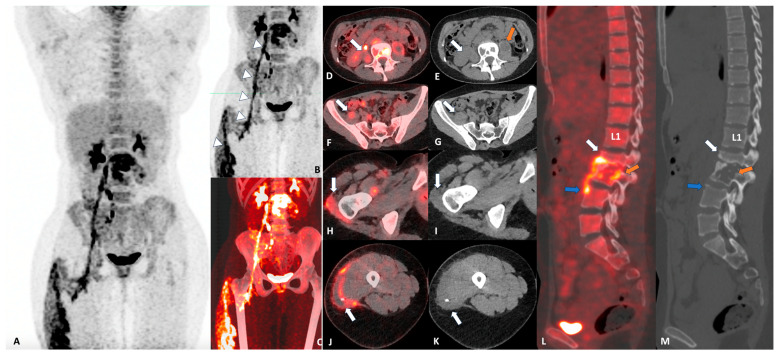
A 24-year-old immunocompetent woman with a history of four months of lower back pain and magnetic resonance imaging (MRI) features suggestive of tuberculous spondylodiscitis of lumbar vertebra 2 and 3 was recruited to the Spinal TB X cohort at the Groote Schuur Hospital of the University of Cape Town (ClinicalTrials.gov Identifier: NCT05610098). As per study protocol, the patient underwent whole-body 2-deoxy-2-[18F]fluoroglucose (FDG) positron emission tomography (PET) computed tomography (PET/CT). PET/CT showed no pathological uptake in either lun’s and there were no pathological changes on CT (A). There was increased uptake in the right psoas muscle extending down anterior to the right hip joint, posterior to and around the trochanteric region of the right femur and into the right thigh, with an SUVmaxbw of 17.0 ((B,C) white triangles; (D,F,H,J) white arrows). On CT, the psoas muscle had an altered density, which spread caudally all the way into the lateral compartment of the thigh ((E,G,I,K), white arrows). Increased uptake was also seen in the left psoas muscle, with an SUVmaxbw of 8.04. A discrete 80 mm long by 40 mm wide psoas collection is noted on the left on CT ((E), orange arrow). In the L2–4 region, vertebral collapse with 50% destruction of L2 ((L,M), white arrow), significant body destruction of L3 ((L,M), orange arrow), and beginning erosion of the anterior body of L4 ((L,M), blue arrow), with an SUVmaxbw of 13, were noted. Subsequently, the patient underwent CT-guided biopsy as per protocol, which revealed drug-sensitive Mycobacterium tuberculosis, and the patient was started on standard tuberculosis treatment for 12 months.
